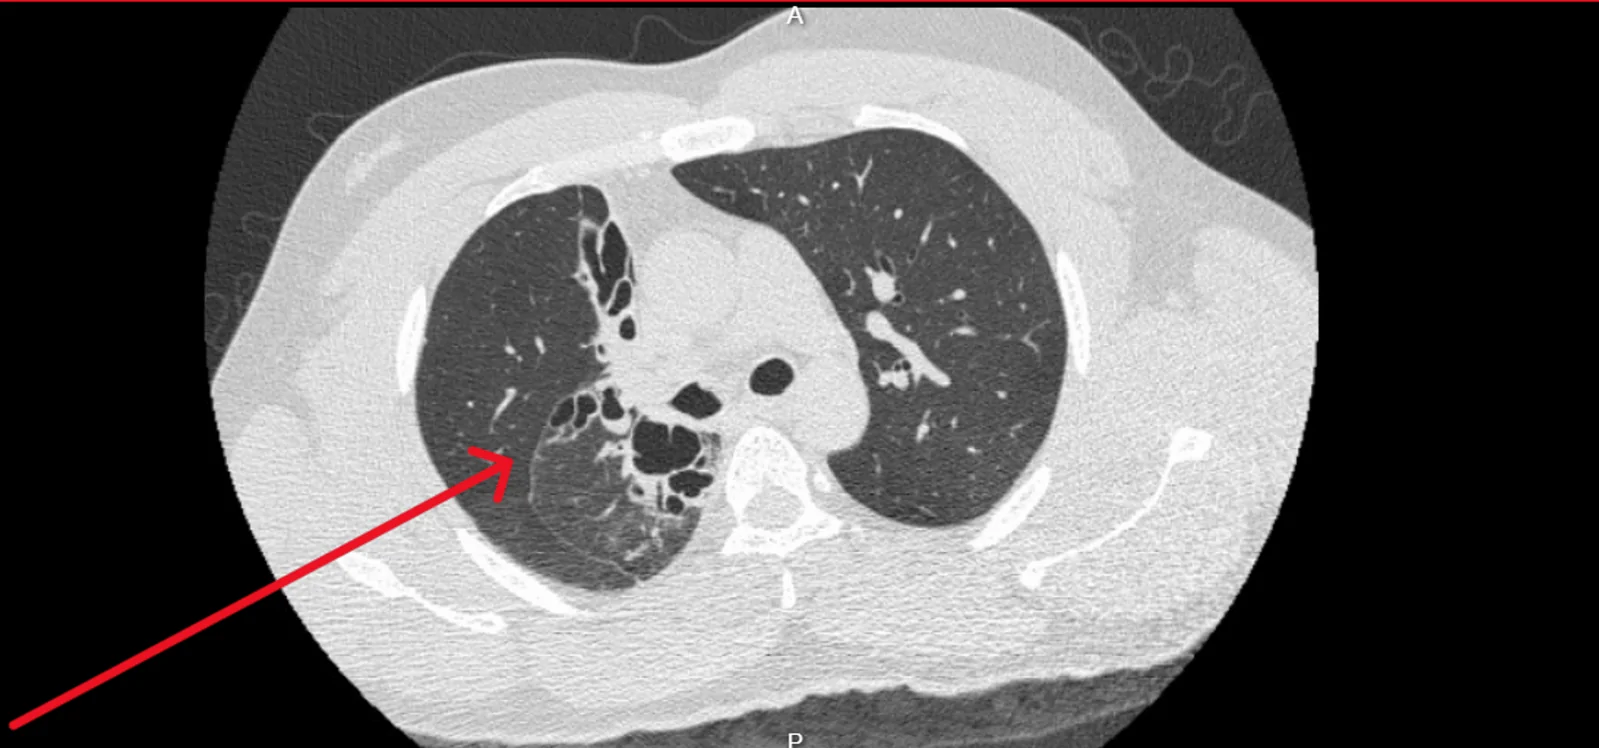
High-resolution CT of the chest demonstrating right-sided cystic bronchiectatic changes with superimposed patchy ground-glass opacities and centrilobular nodules, consistent with an acute infective process.
